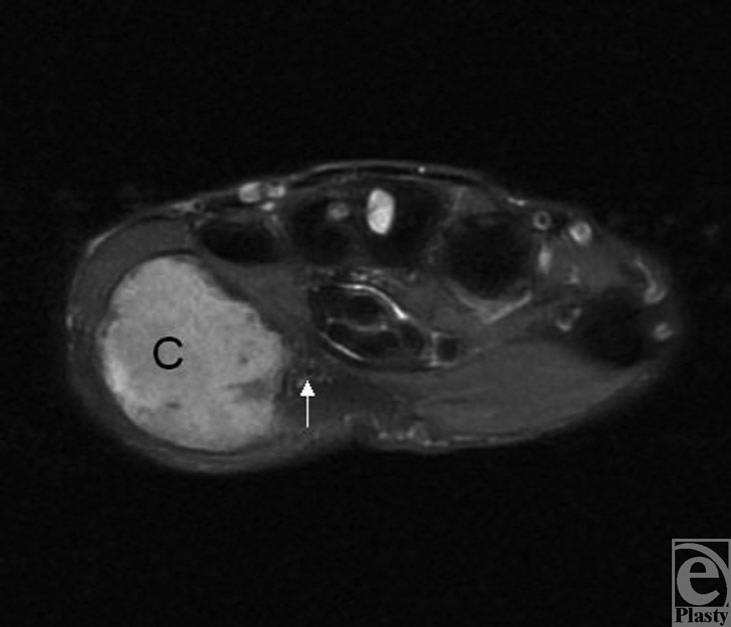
Axial T2-weighted magnetic resonance imaging scan showing loculated lesion (C) of high signal intensity lying in the hypothenar eminence adjacent to Guyon's canal (white arrow).
